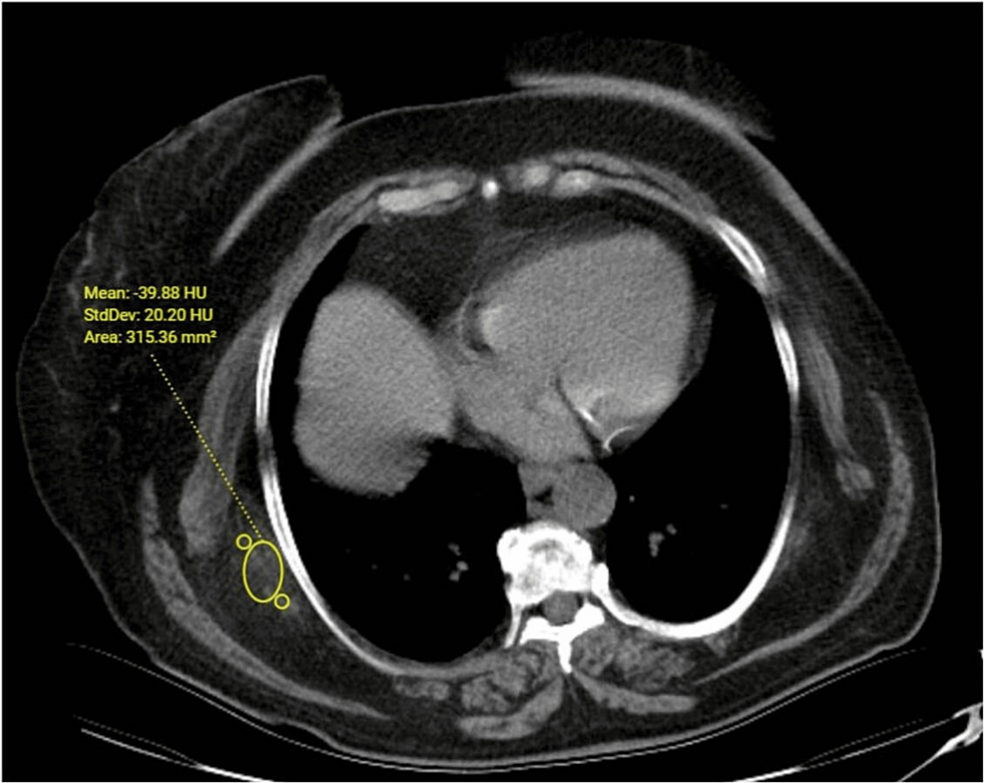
Axial non-contrast CT image. Density was measured to be -39 HU for right-side ED in a 68-year-old female patient.
